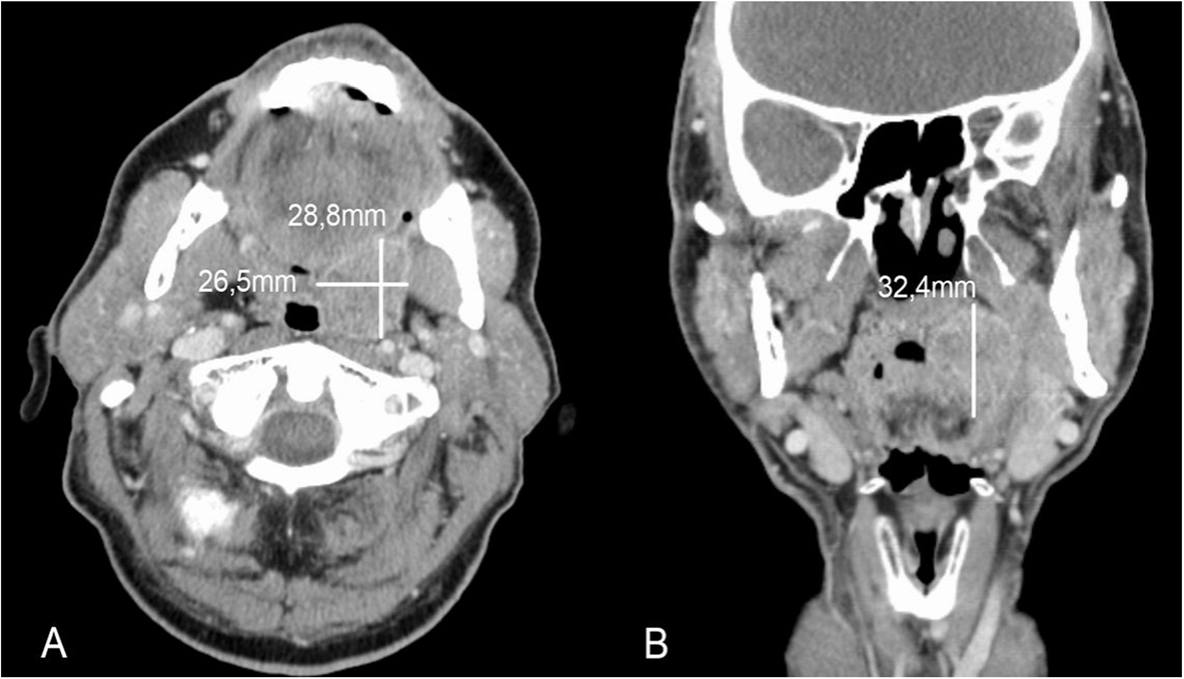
“Maximum tumor diameter assessment in axial and coronal contrast enhanced diagnostic CT scan”. 70 year old male patient suffering from cT2 cN0 cM0 oropharyngeal squamous cell carcinoma. a Axial section with maximum anterior-posterior and medio-lateral tumor diameters (white lines). b Coronal section with maximum cranio-caudal tumor diameter
